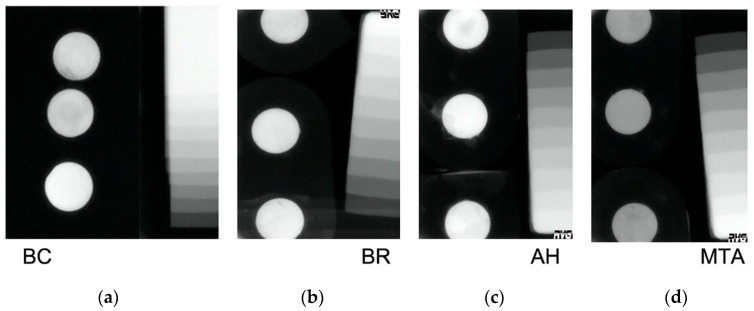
Digital x-rays of (a) BC sealer; (b) BR sealer; (c) AH sealer; (d) MTA sealer.
